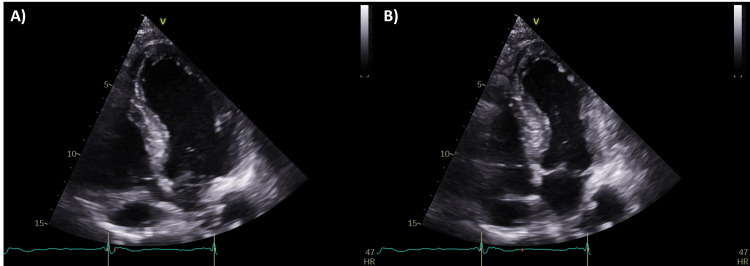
Snapshots from apical four-chamber transthoracic echocardiogram (TTE) at end diastole (A) and end systole (B), demonstrating good basal contraction with impaired mid-apical function.
